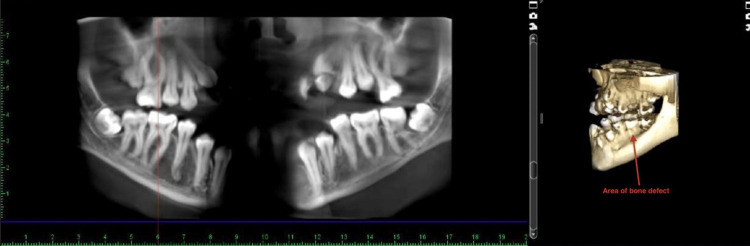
Cone-beam computed tomography image of grade III furcation involvement
